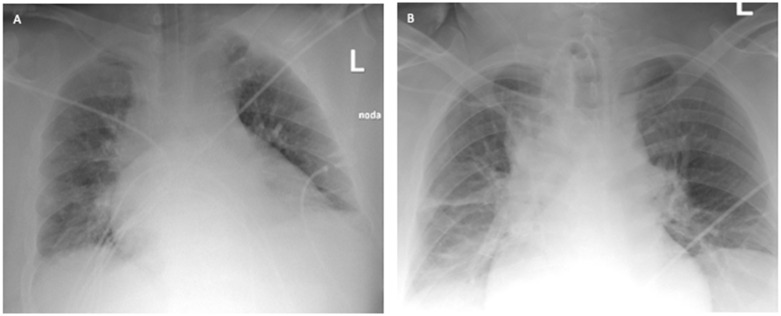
Chest X-ray: (A) after admission to the ICU; (B) 2 days after starting VV ECMO treatment (Case 2).
